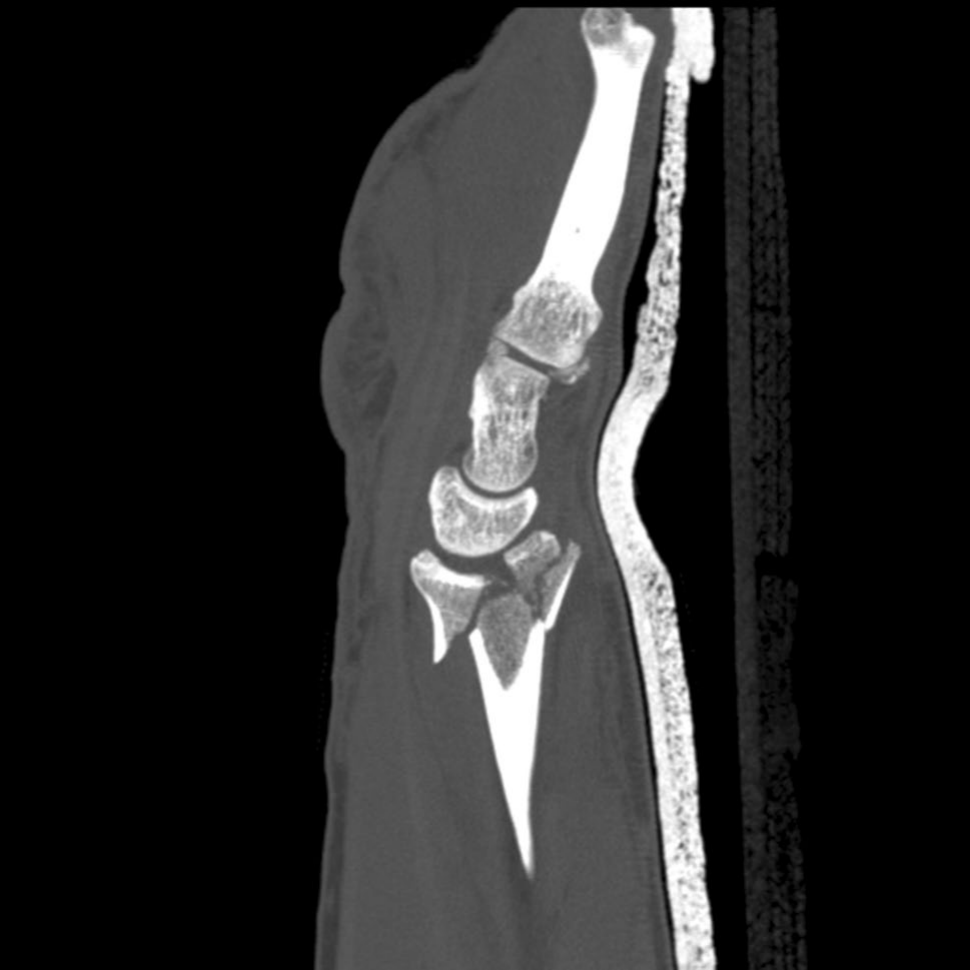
CT scan of a lateral view of the intermediate column showing the volar fragment of the intermediate column, a fracture gap and step of more than 2 mm and the volar fragment additionally toppled
